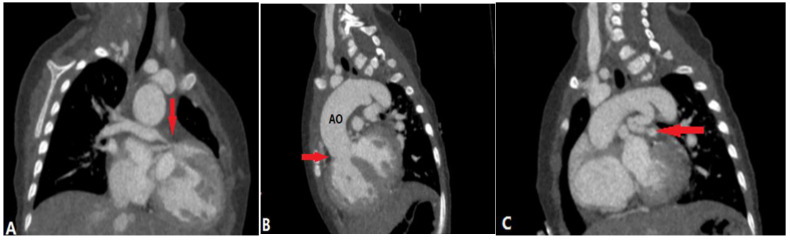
Pulmonary artery atresia with ventricular septal defect. (A): Coronal CT angiography shows that the main pulmonary artery is atretic and the right pulmonary artery is slender (arrow). (B): Oblique coronal CT shows outlet ventricular septal defect (arrow) and overriding aorta (AO). (C): Oblique coronal CT demonstrates patent ductus arteriosus (arrow) originating from aorta to left pulmonary artery.
